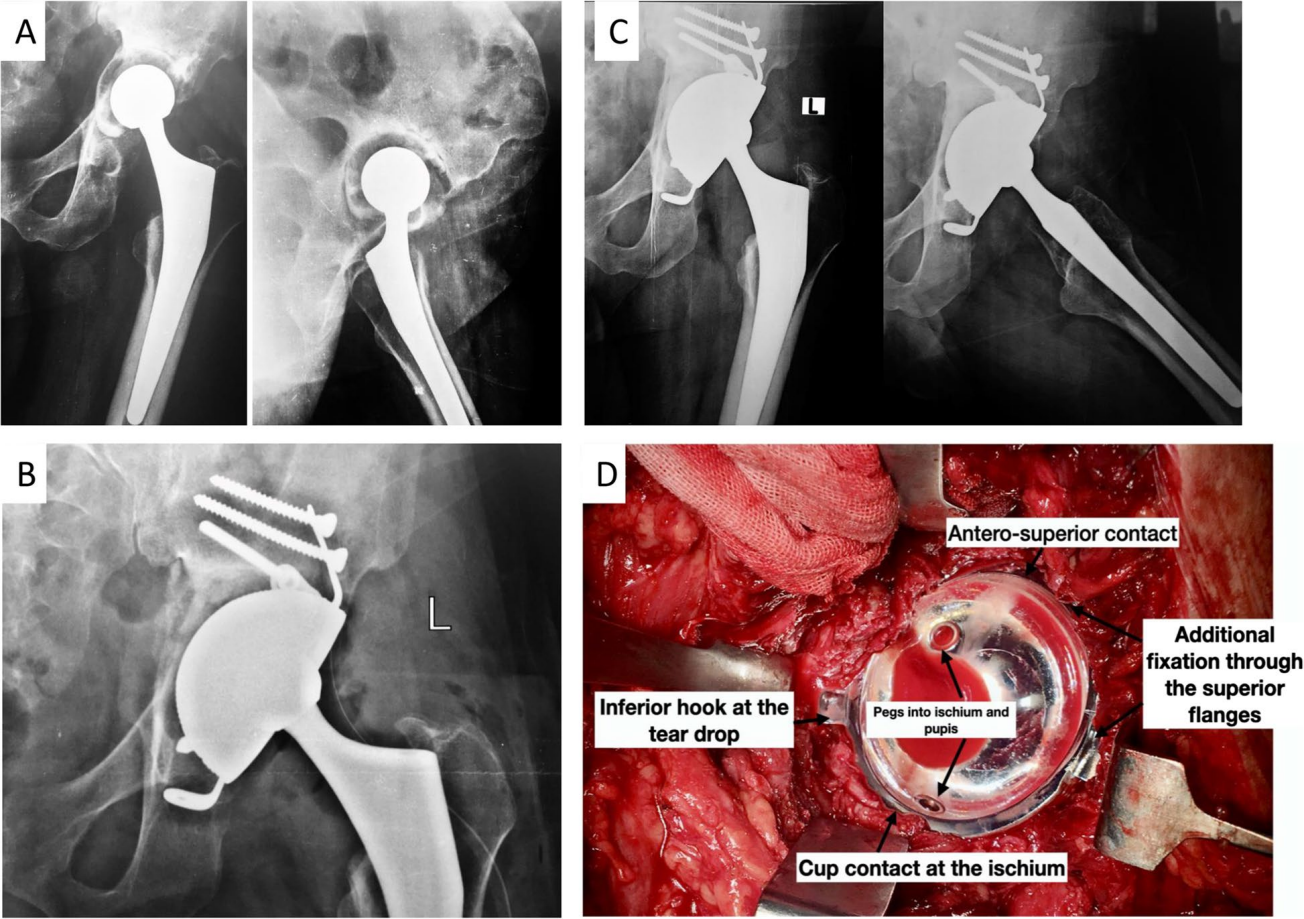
A Preoperative x-ray of a loose cemented cup with proximal migration > 20 mm and medial defect Paprosky IIIB. B x-ray at 3 months posts operative showing cup position and medial graft. C 3 years post-operative with cup stability, restoration of the hip center of rotation, and graft incorporation. D Intraoperative photo demonstrating Coptos cups rim contact in addition to intraarticular and extra-articular additional fixation
